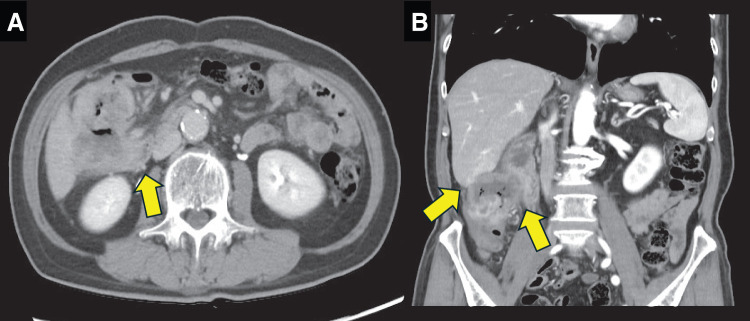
(A) Axial and (B) coronal computed tomography showed a 12-cm mass lesion with wall thickening in the ascending colon invading the second portion of the duodenum, and a small extent of invasion into the liver was also observed (arrows).
